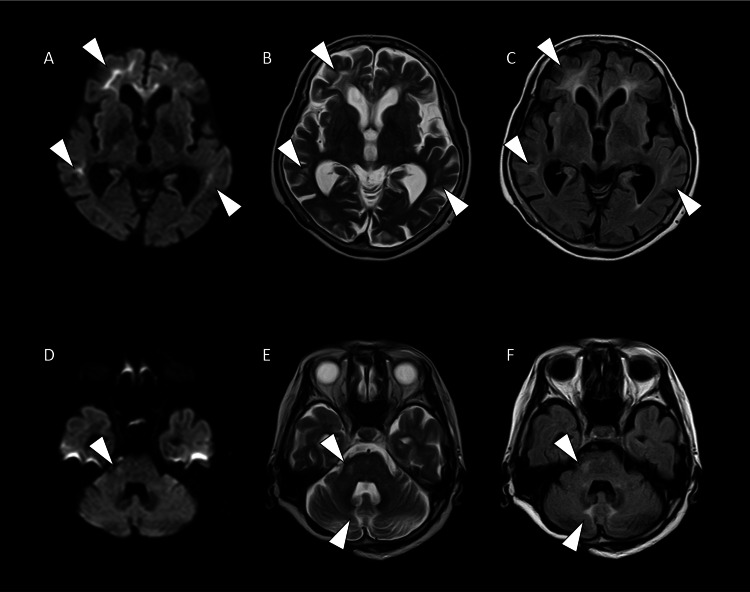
Brain MRI at diagnosis DWI: diffusion-weighted imaging; T2WI: T2-weighted imaging; FLAIR: fluid attenuated inversion recovery DWI (A,D) showing a ribbonlike hyperintensity (arrowhead) at the right frontal and temporal corticomedullary junctions, along with a hyperintense lesion (arrowhead) in the middle cerebellar peduncle. T2WI (B,E) and FLAIR (C,F) images showing hyperintense lesions (arrowhead) in the right frontal and temporal corticomedullary junctions, middle cerebellar peduncle, and paravermian cerebellar region. Compared with the images obtained at the initial visit (four years earlier), these abnormalities were more conspicuous
